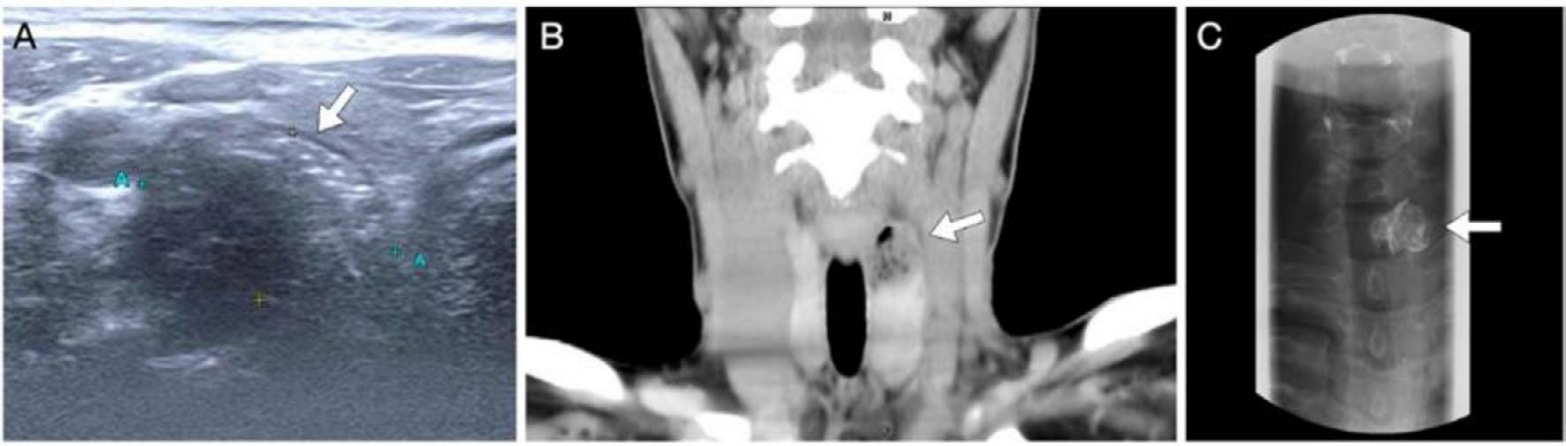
Imaging findings of Killian–Jamieson diverticulum mimicking a thyroid nodule. (A) Ultrasonography showing a 2.1‐cm hypoechoic lesion with punctate echogenic foci (arrow) in the upper pole of the left thyroid lobe, initially suspicious for malignancy. (B) Coronal computed tomography scan demonstrating intralesional air within a paracervical esophageal lesion (arrow). (C) Barium esophagography confirming a left anterolateral cervical esophageal outpouching (arrow), consistent with Killian–Jamieson diverticulum.
